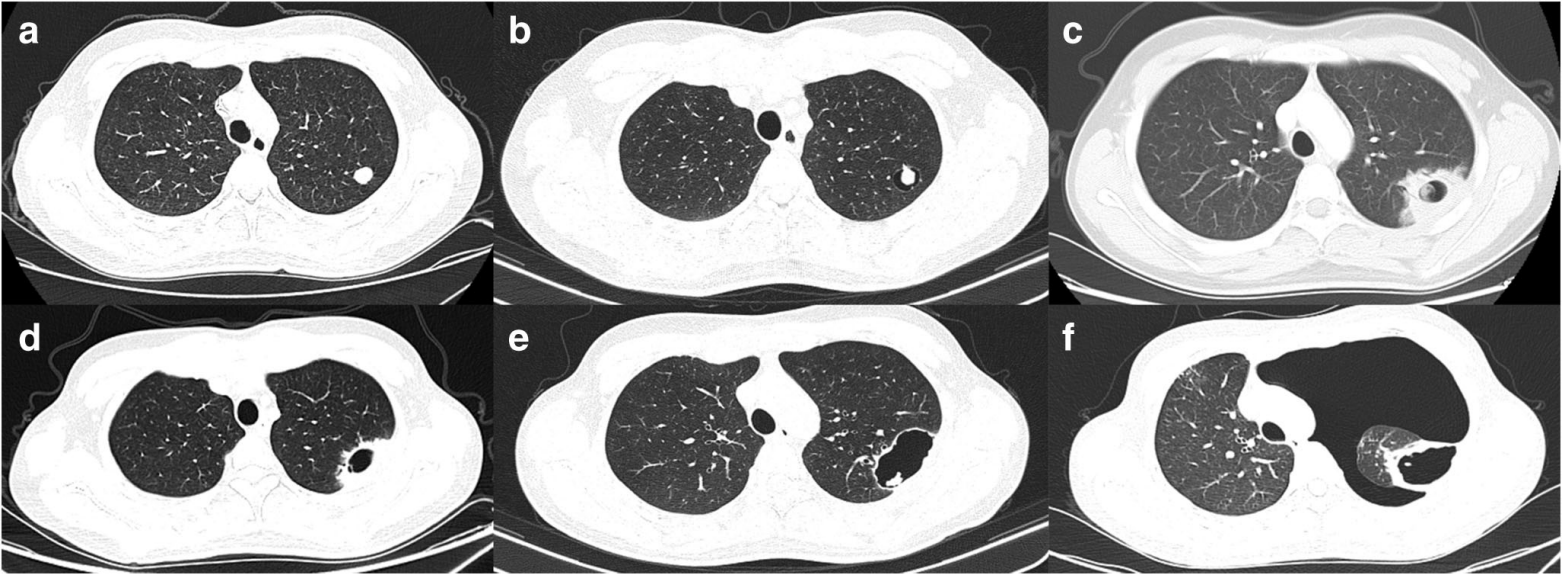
Typical development of pneumothorax after apatinib treatment in an osteosarcoma patient. Computed tomography scans were obtained a at treatment initiation, b 1 month, c 2 months, d 3 months, e 5 months, and f 6 months after treatment
